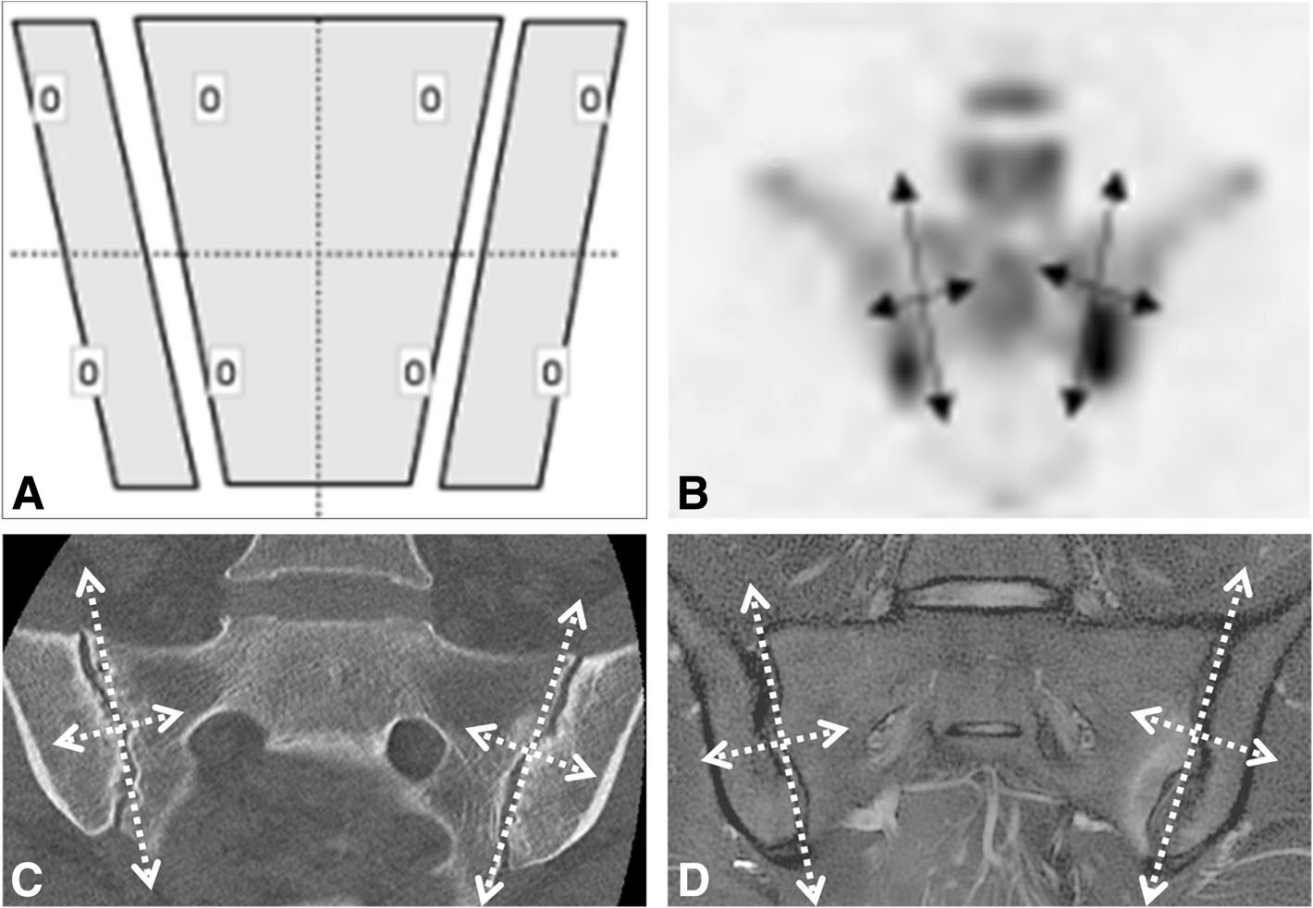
Methodology of the semi-quantitative evaluation of the SIJ using for quadrants, based on the SPARCC MRI SIJ inflammation score on the cartilaginous part of the SIJ (a). Application on PET/CT (b), CT scan (c), and MRI (d)
